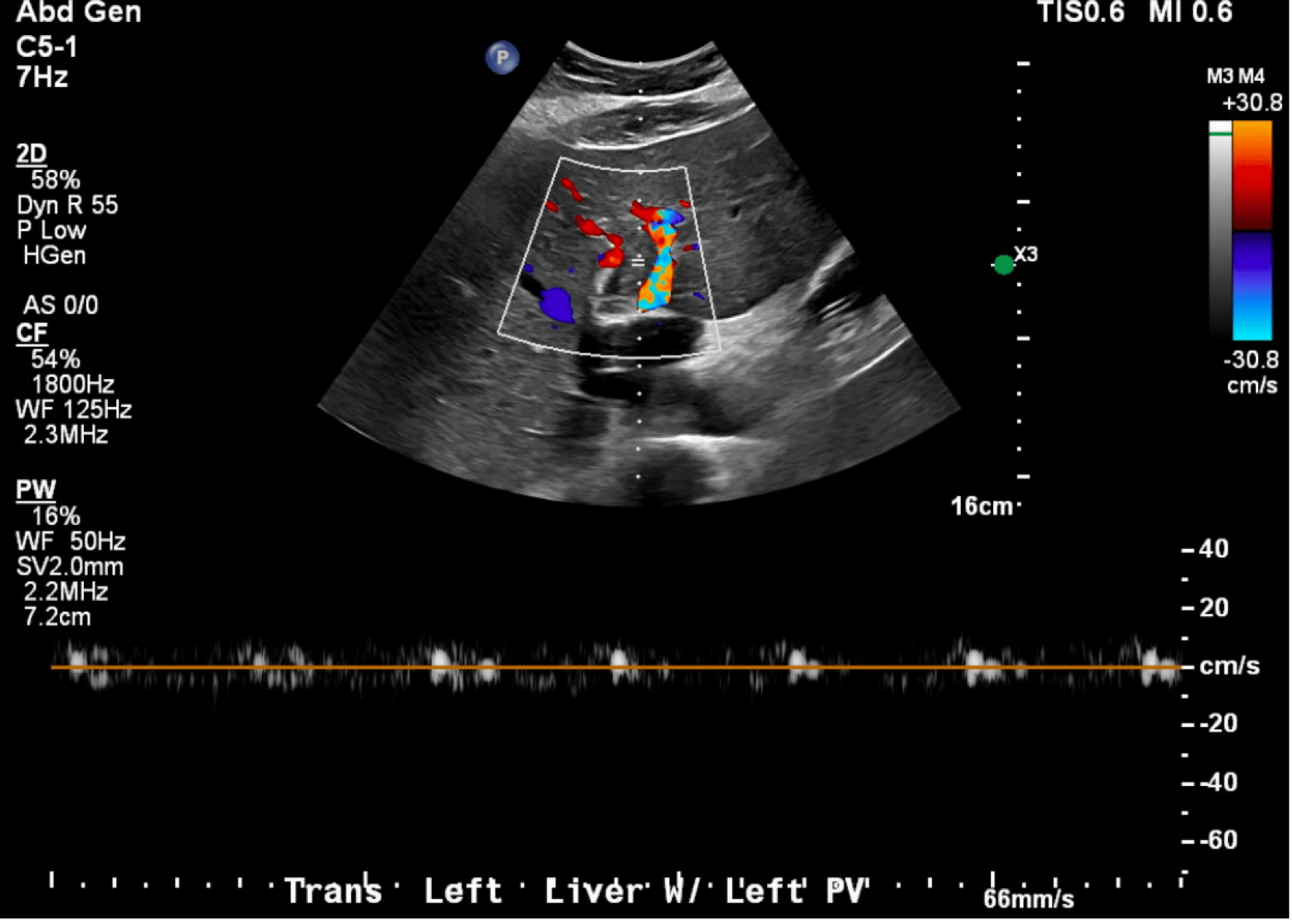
Abdominal ultrasound of the left portal vein. Ultrasound demonstrated a patent right portal vein with normal flow direction, as well as thrombosis of the left portal vein without an associated mass lesion.
